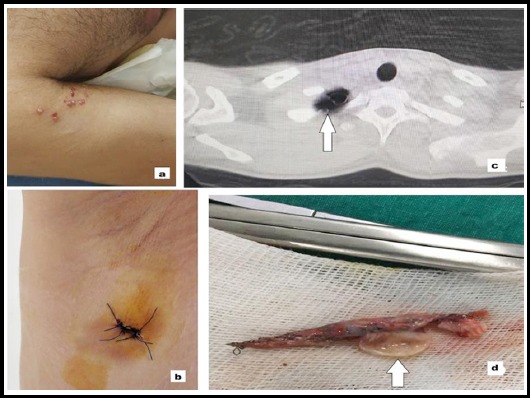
Varicella zoster skin lesion, 1b: The uniportal incision is made on the 5th intercostals space, anterior axillary line. (2,5 cm), 1c: CT scan of the chest showing bullae (arrows) in the apex of the lung, 1d: Thoracoscopic resection for bleb disease.
